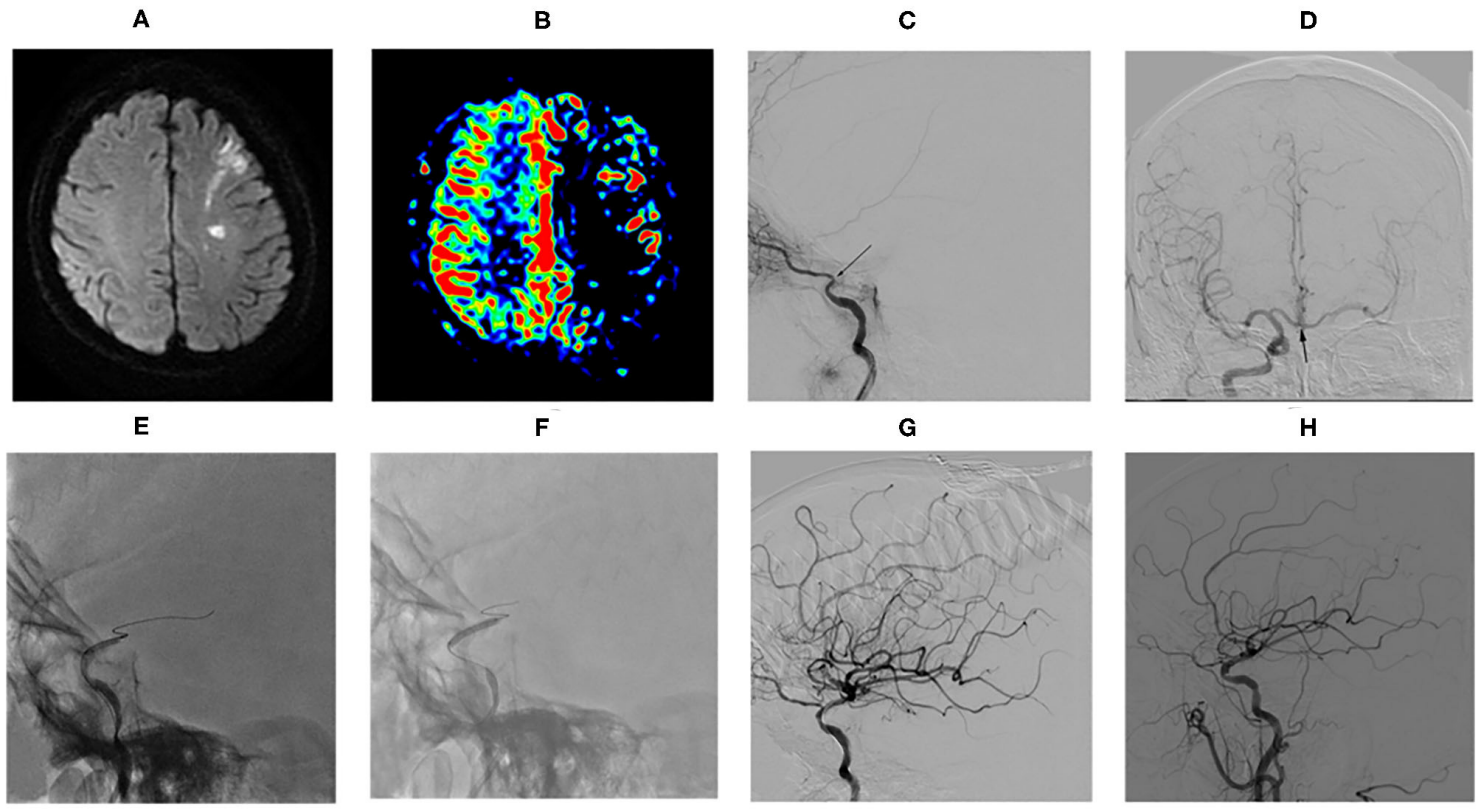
Example of drug-coated balloon (DCB) dilatation for nonacute sICATO and follow-up. (A) MRI revealed left watershed infarction. (B) ASL showed left cerebral hemispheric hypoperfusion in the ICAO territory. (C) DSA confirmed the left internal carotid artery terminus occlusion ipilaterally to the infarcts (arrow). (D) DSA showed anterior communicating arteries (arrow). (E) Predilatation with a conventional balloon. (F) DCB dilatation after predilatation. (G) Angiographic result after the procedure. (H) Angiographic result at follow-up of 3.0 months.
